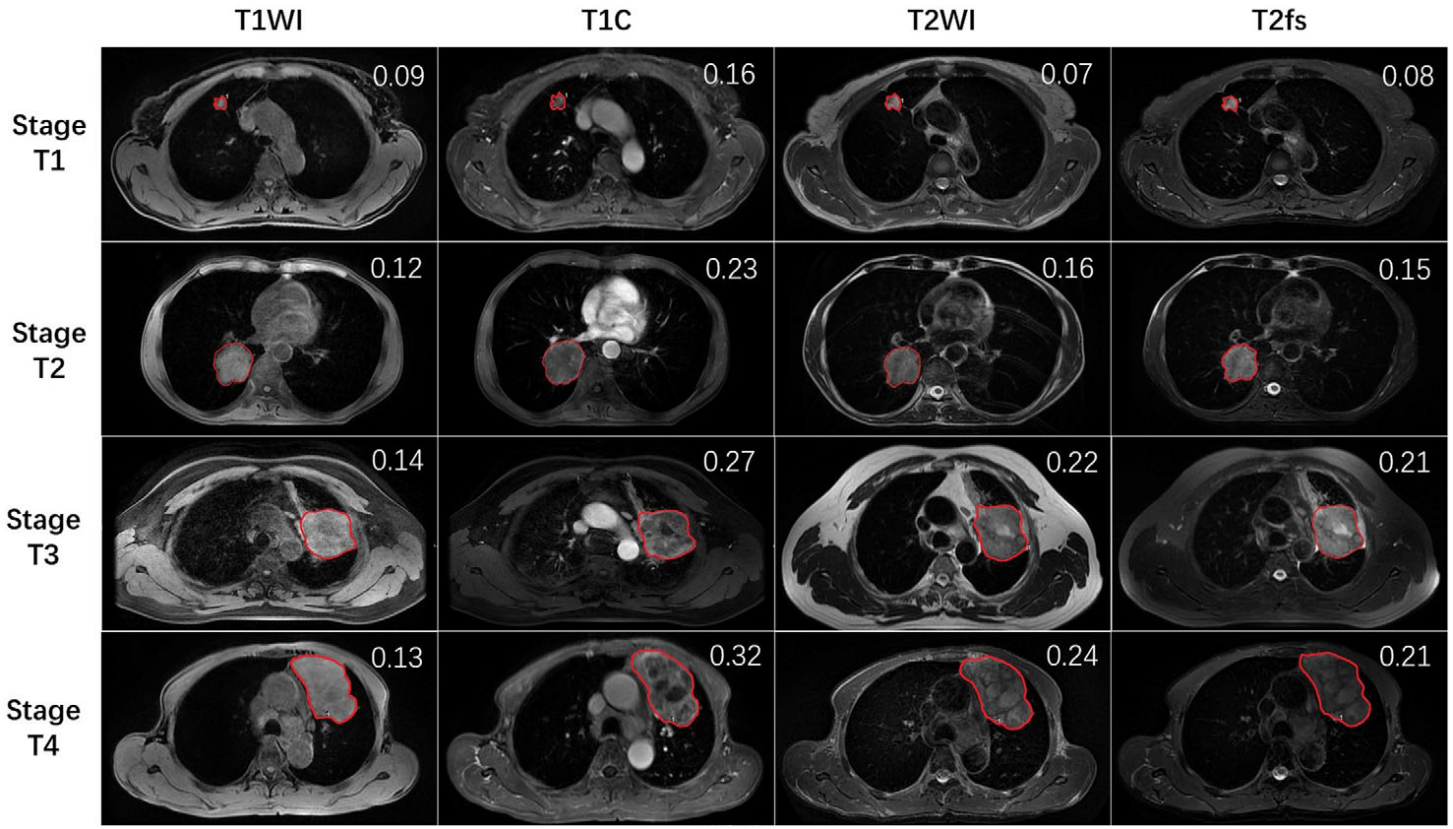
The representative MR images of stage T1–4 patients. Red lines delineate the ROI, which was delineated on the largest cross-sectional slice of primary lung tumor. The SD/mean value was shown on the right upper top of each image.
